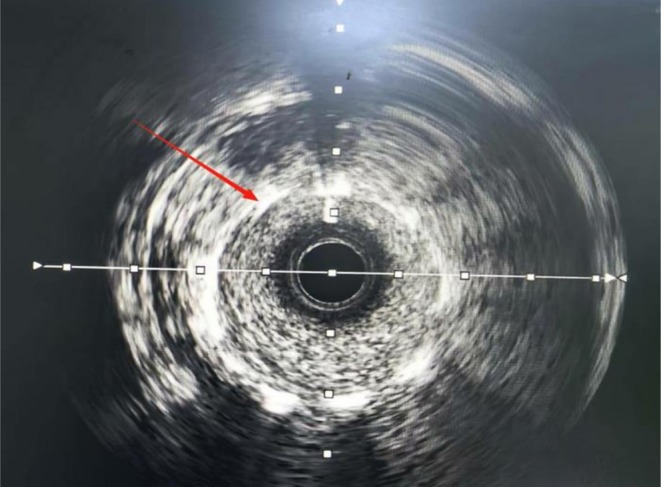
Coronary artery intravascular ultrasound image showing optimal stent expansion and good apposition to the vessel wall following intervention.
